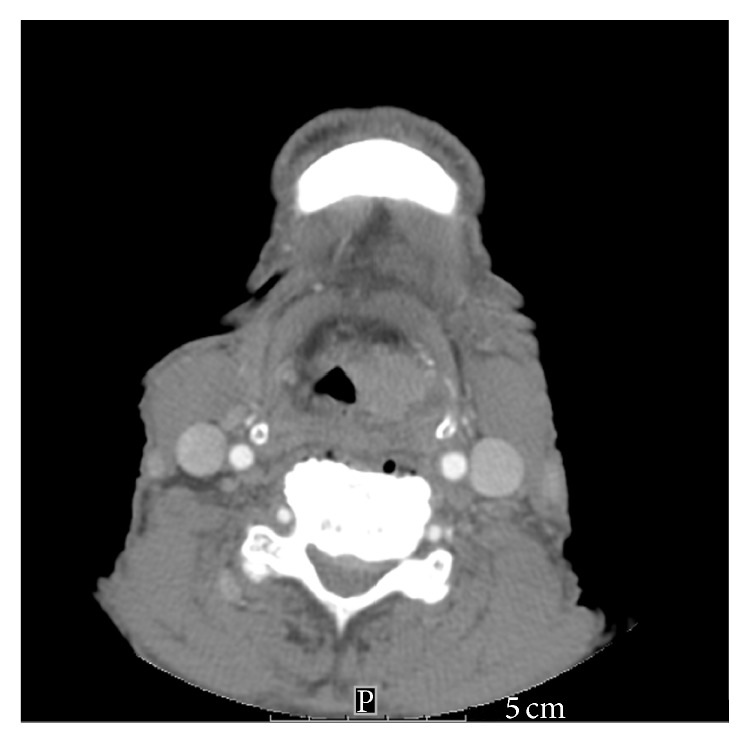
Neck CT showing patient's left glottis tumor.
